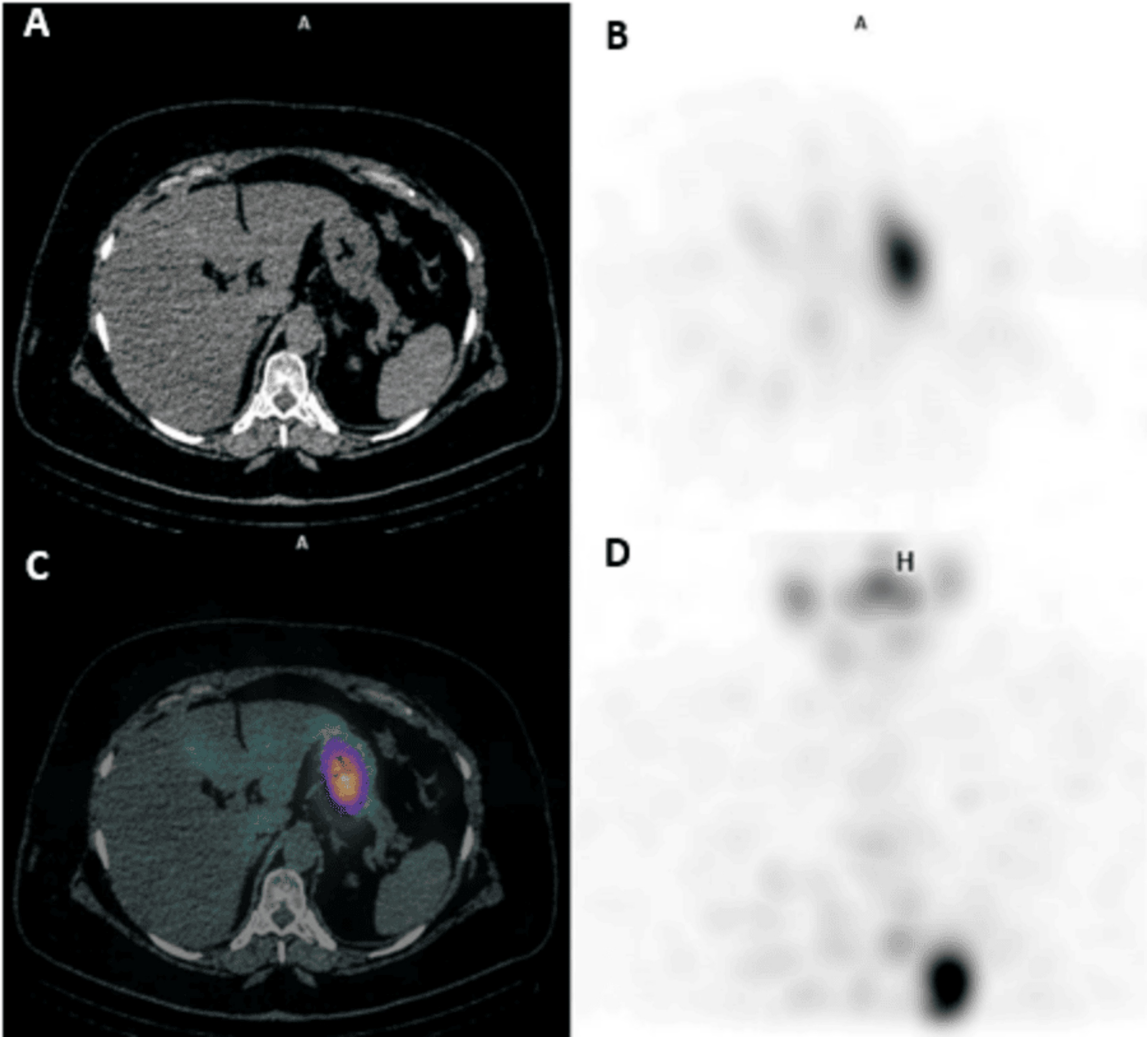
Diagnostic whole-body RAI SPECT/CT scan (third RAI dose). Diagnostic whole-body RAI SPECT/CT scan done six months after the third RAI dose shows complete resolution of the RAI uptake within hepatic segment VII in SPECT, fused, and MIP images (B, C, and D respectively). RAI, radioactive iodine; SPECT/CT, single photon emission computed tomography/computed tomography; MIP, maximum intensity projection
